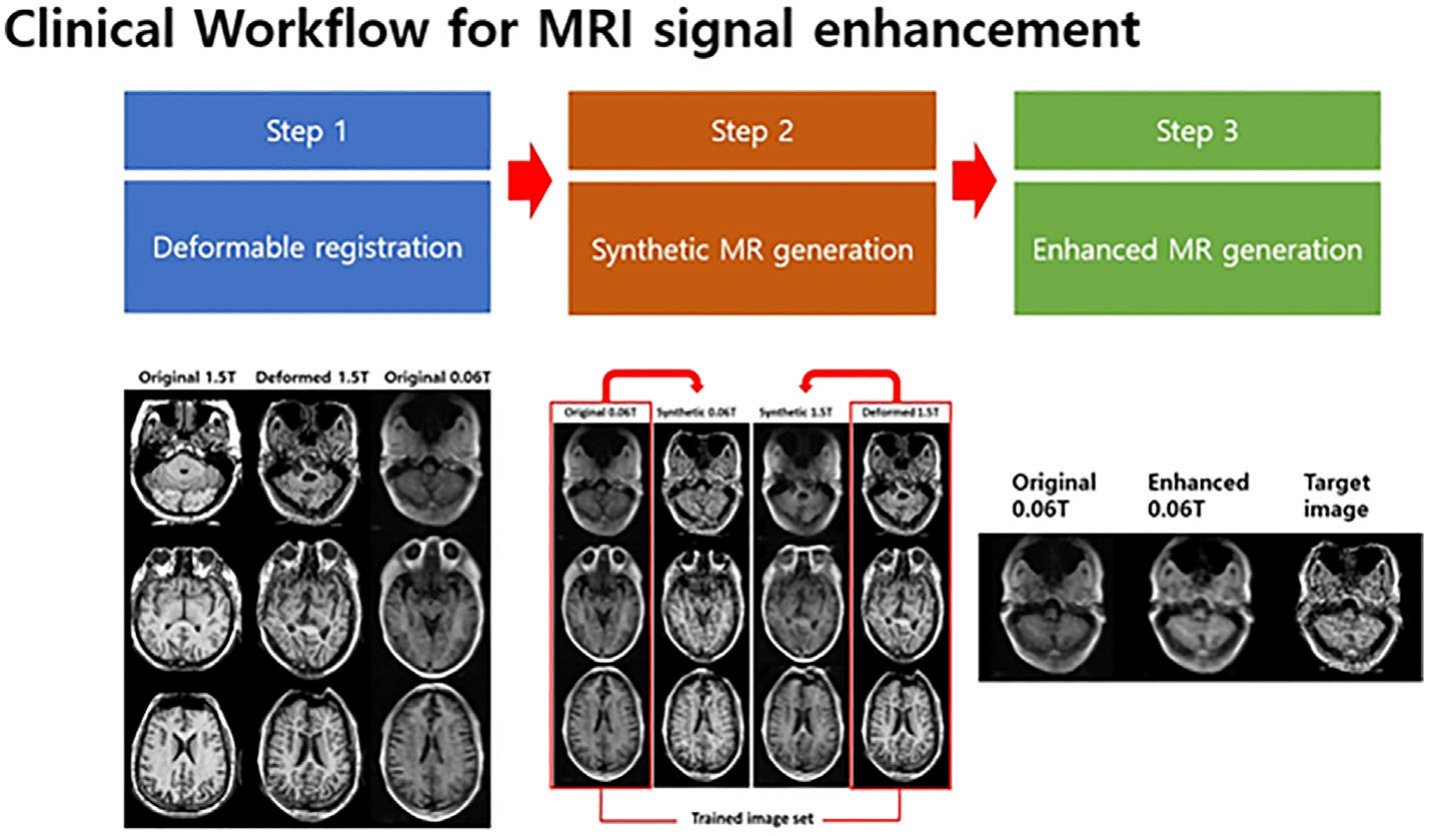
Clinical flow for enhancement of unpaired MRI set.
